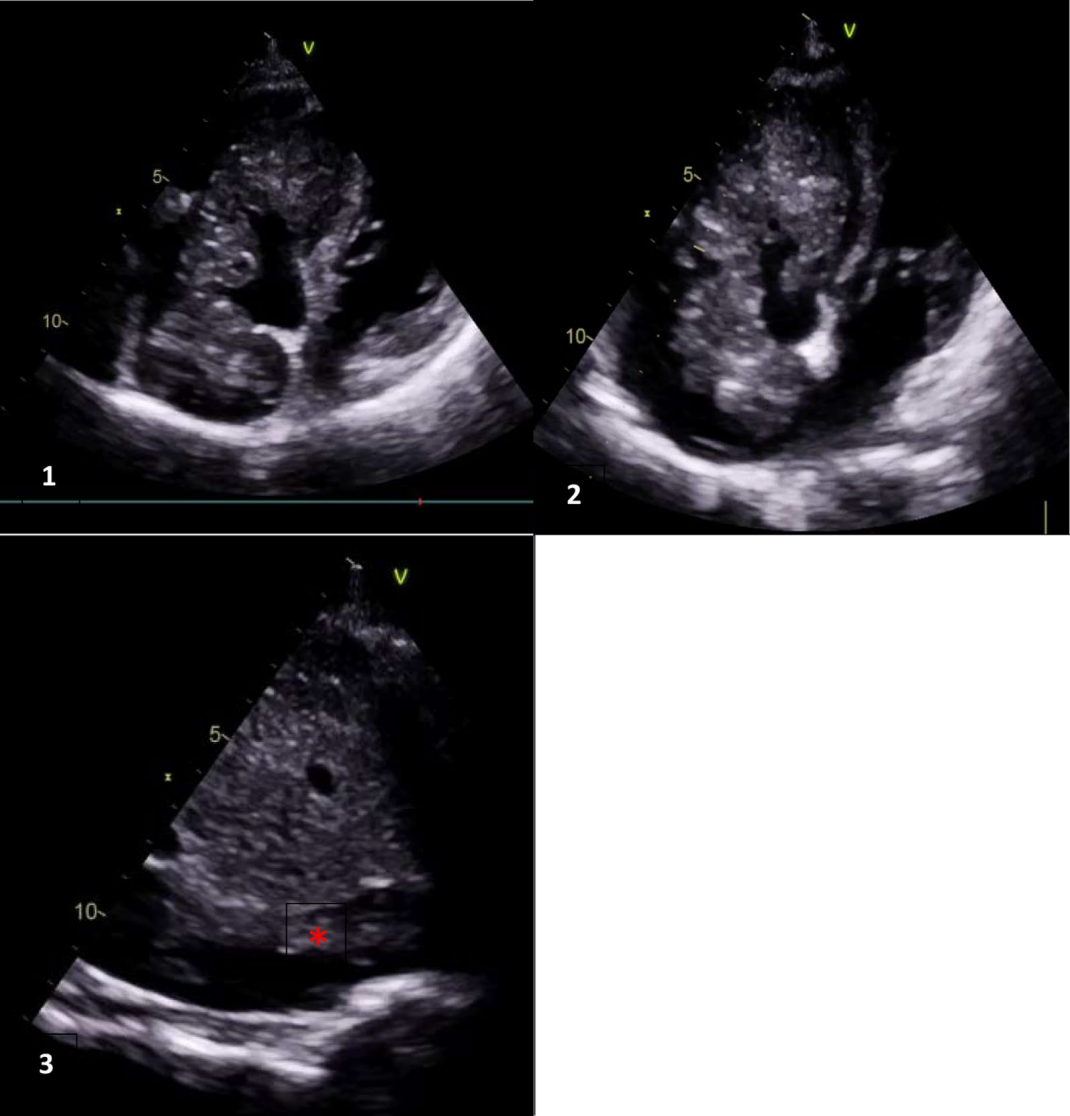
Transthoracic echocardiography: 1–2 4 chambers view: polylobed echogenic mass, mildly calcified hanging between the right atrium and the right ventricle through the tricuspid valve, measuring 70 mm along its length, obstructing intermittently the tricuspid flow. 3 Sub costal view, Red Asterix: tumoral extension through the inferior vena casa
